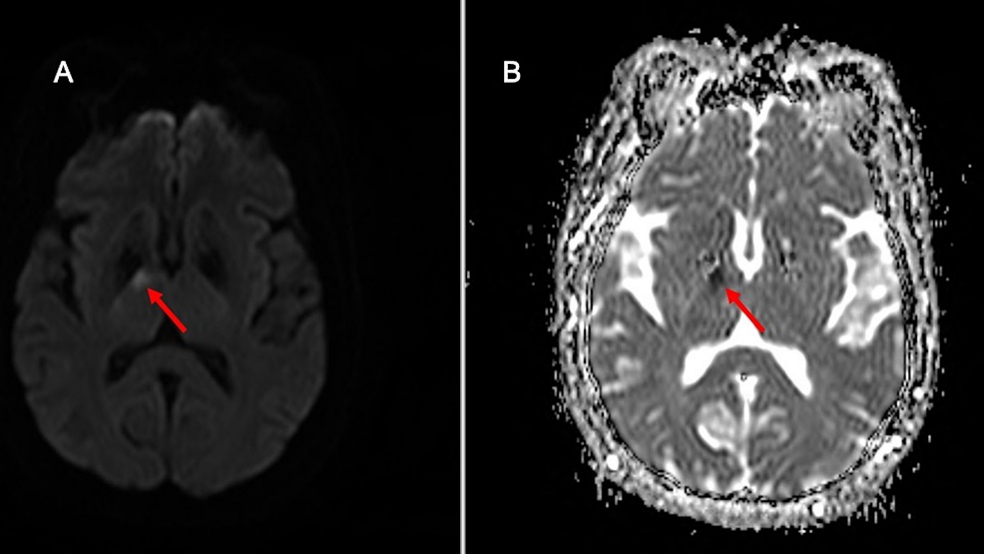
Axial (A) DWI with corresponding (B) ADC map of the brain confirm the area of ischemia in the anterior right thalamus as depicted by the hyper-intense and concurrent hypo-intense signal on the DWI and ADC images, respectively (red arrows). DWI, diffusion weighted image; ADC, apparent diffusion coefficient
